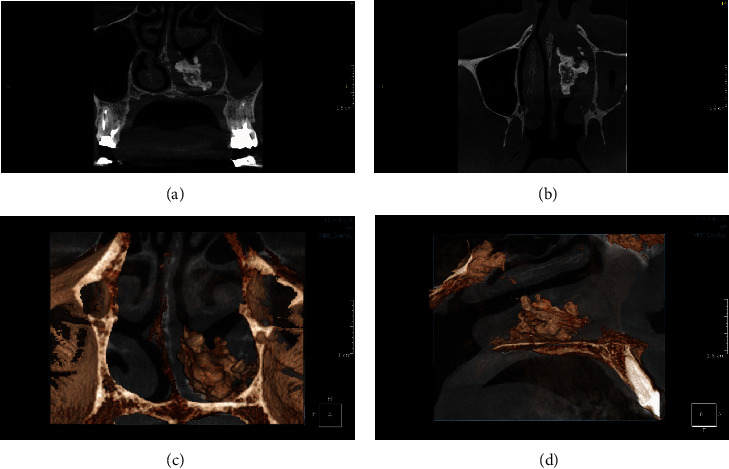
CBCT images of nasal fossa. Coronal (a), axial (b), and multiplanar reformat images show an irregularly large heterogenous calcified mass in the inferior meatus of the left nasal cavity. Note the adjacent mucosal thickening. Coronal (c) and sagittal (d) 3D reformation images better show the space occupying rhinolith and slight deviation of nasal septum to the right side.
